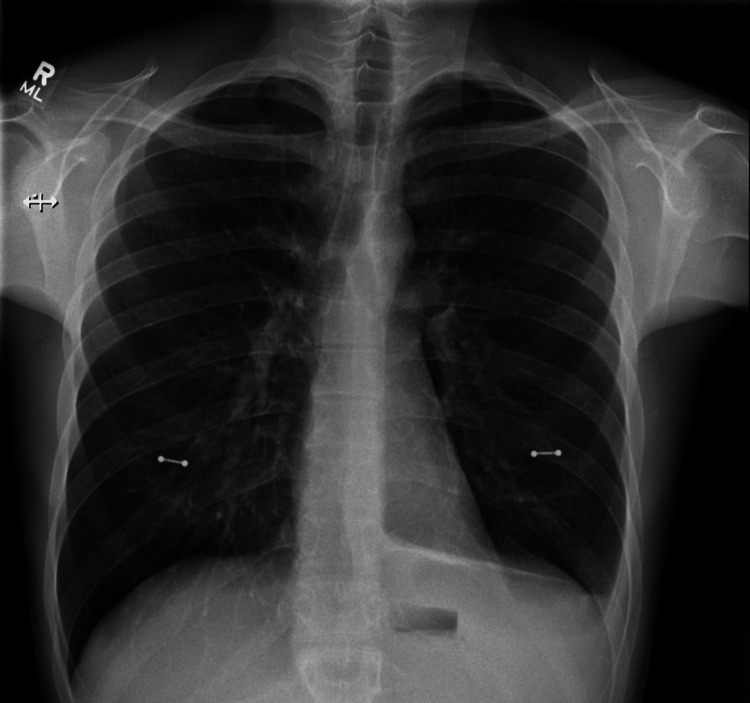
Chest X-ray showing spontaneous near resolution of left-sided pleural effusion.
